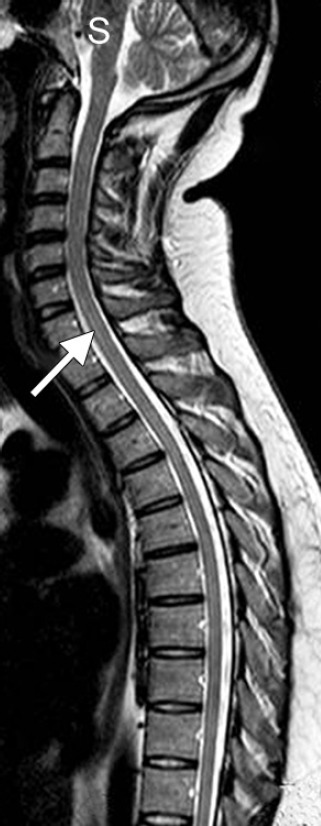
MRI brain and spine showing T1 hyperintensity in cervicodorsal area
